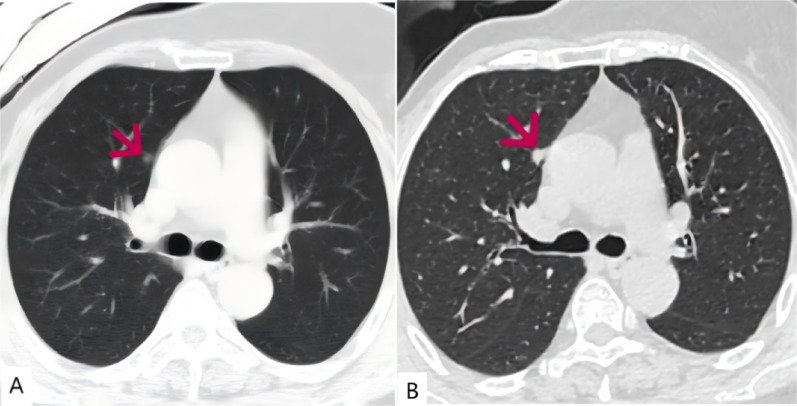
CT scan of pulmonary nodule: (A) initial nodule size 4 mm. (B) follow-up CT showed nodule 9 mm
